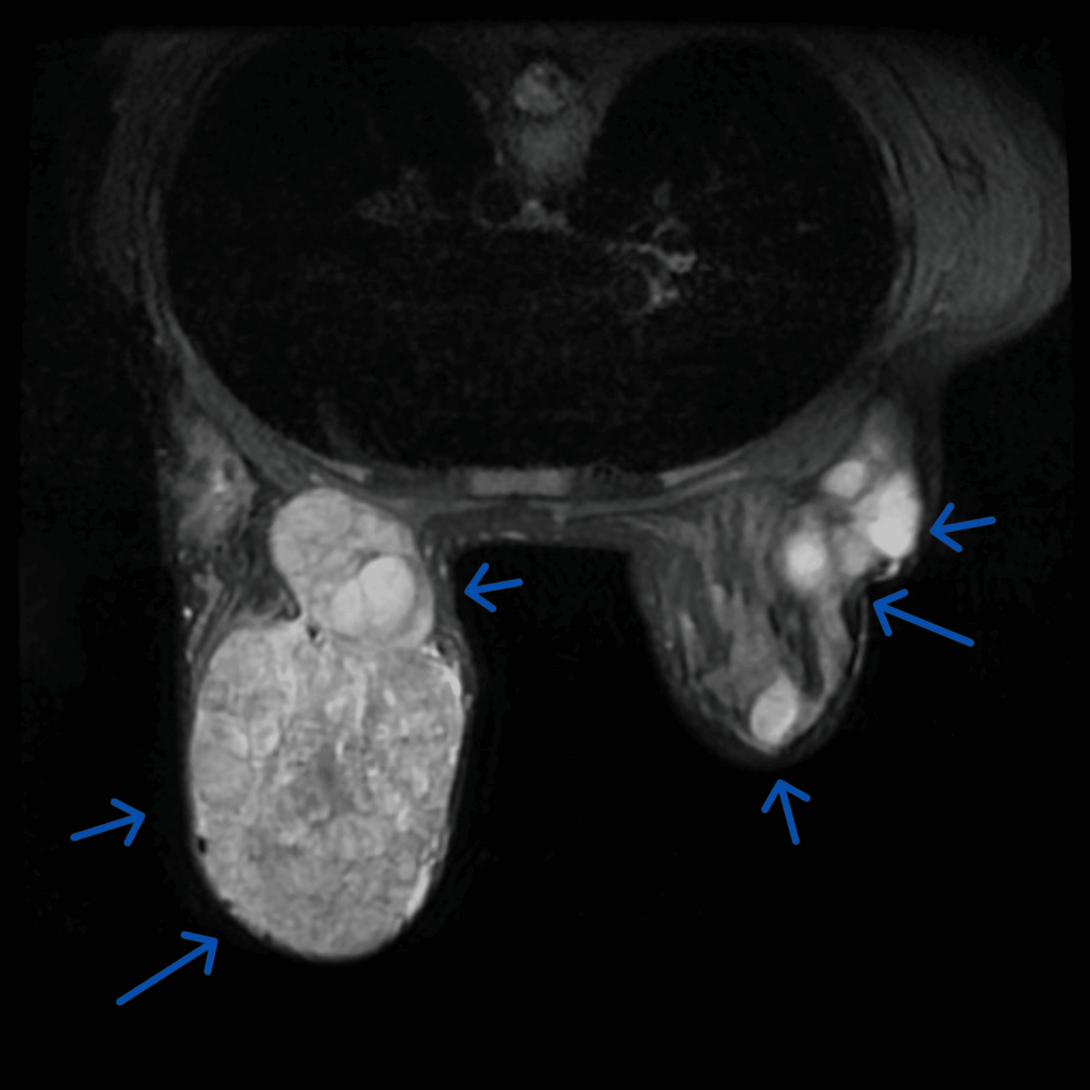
MRI image of both breasts The image shows multiple fibroadenomas occupying the whole breast tissue. Arrows show bilateral huge breast masses occupying and compressing the rest of the breast tissue.
